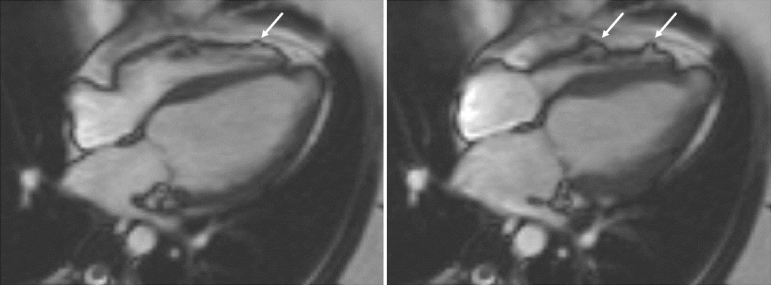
Four-chamber view in diastole (left panel) and systole (right panel). The left ventricle has an apical aneurysm and multiple aneurysms and areas of dyskinesia in the right ventricular free wall are seen (arrowed). This cardiovascular magnetic resonance study had been undertaken 3 months following the initial presentation and the ejection was only mildly impaired, showing a significant improvement when compared with the echocardiogram from 3 months previously.
